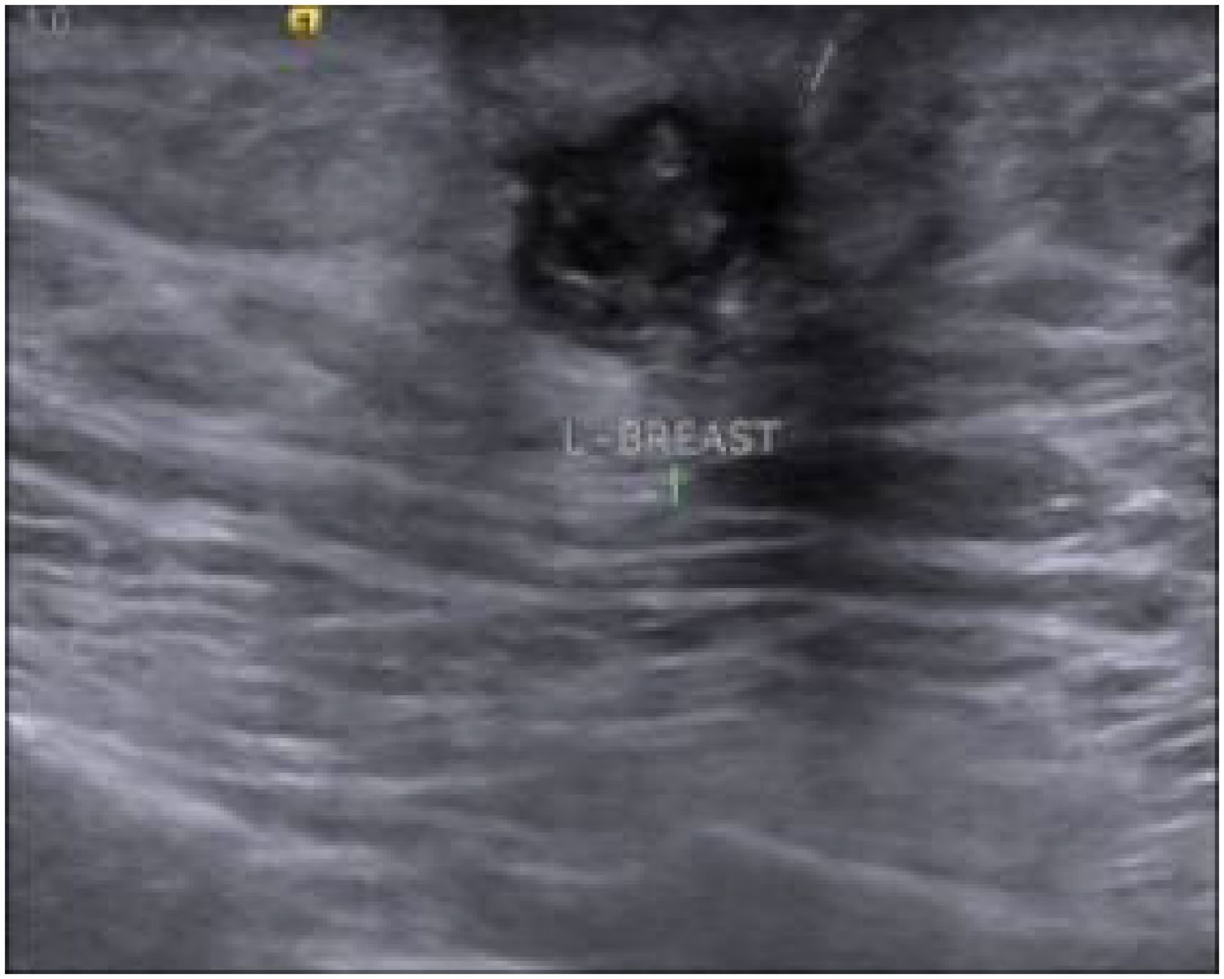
Color Doppler ultrasound of the left breast. A 10 × 8 mm hypoechoic mass at the left nipple root demonstrated irregular spiculated margins (characteristic of malignancy), heterogeneous internal echotexture with focal hyperechogenicity (arrow), and well-defined borders with indistinct posterior acoustic shadowing.
